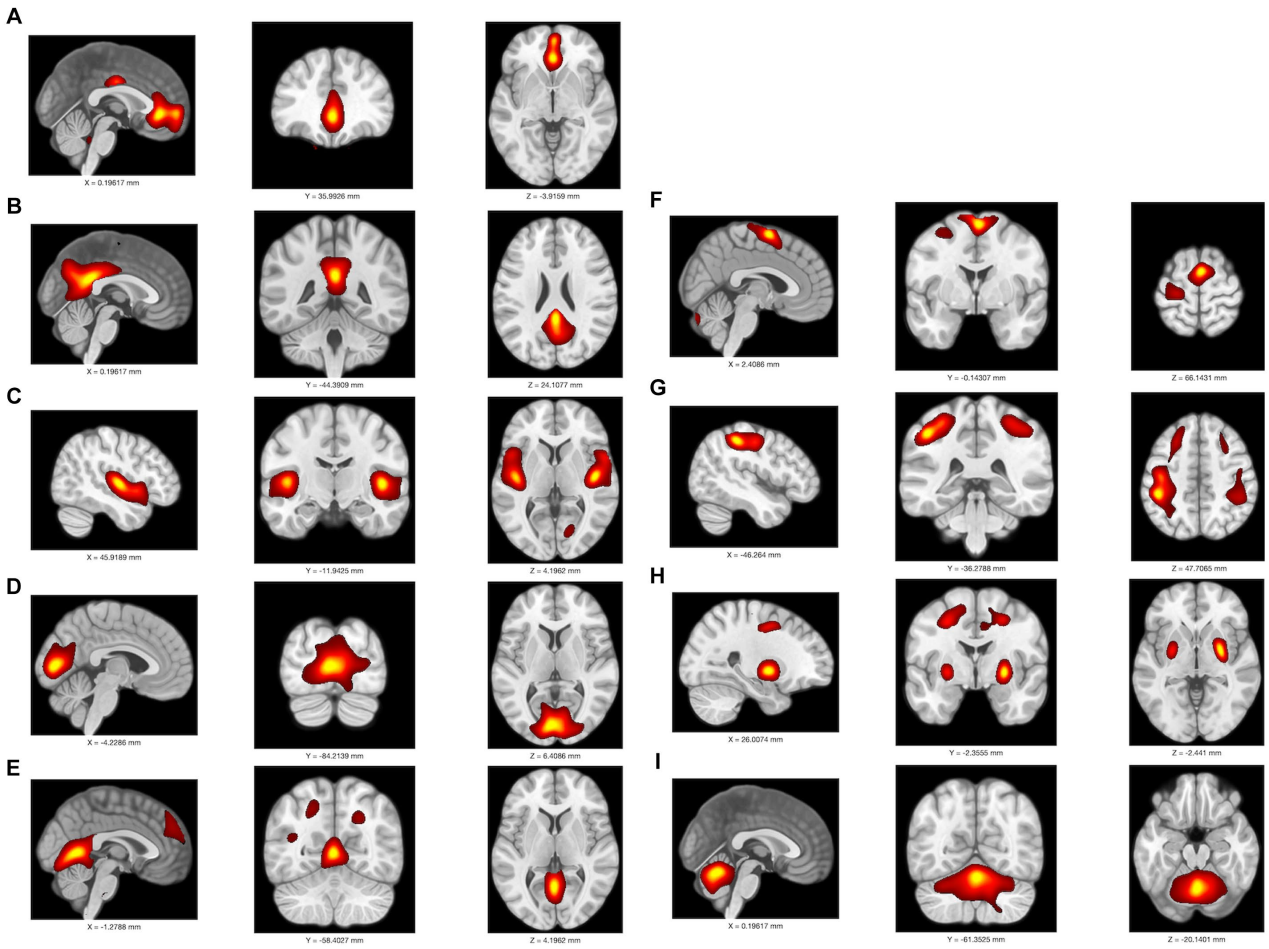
An overlay is displayed of the independent component map at a threshold of *z* > 2.0 on a T1 template in MNI space in F-18 FDG-PET; it includes (A) anterior DMN, (B) posterior DMN, (C) auditory network; AUN, (D–E) primary visual network; pVIS, (F) somatosensory network; SMN, (G) executive control network; ECN, (H) basal ganglia network; BGN, (I) cerebellar network; CBN.
